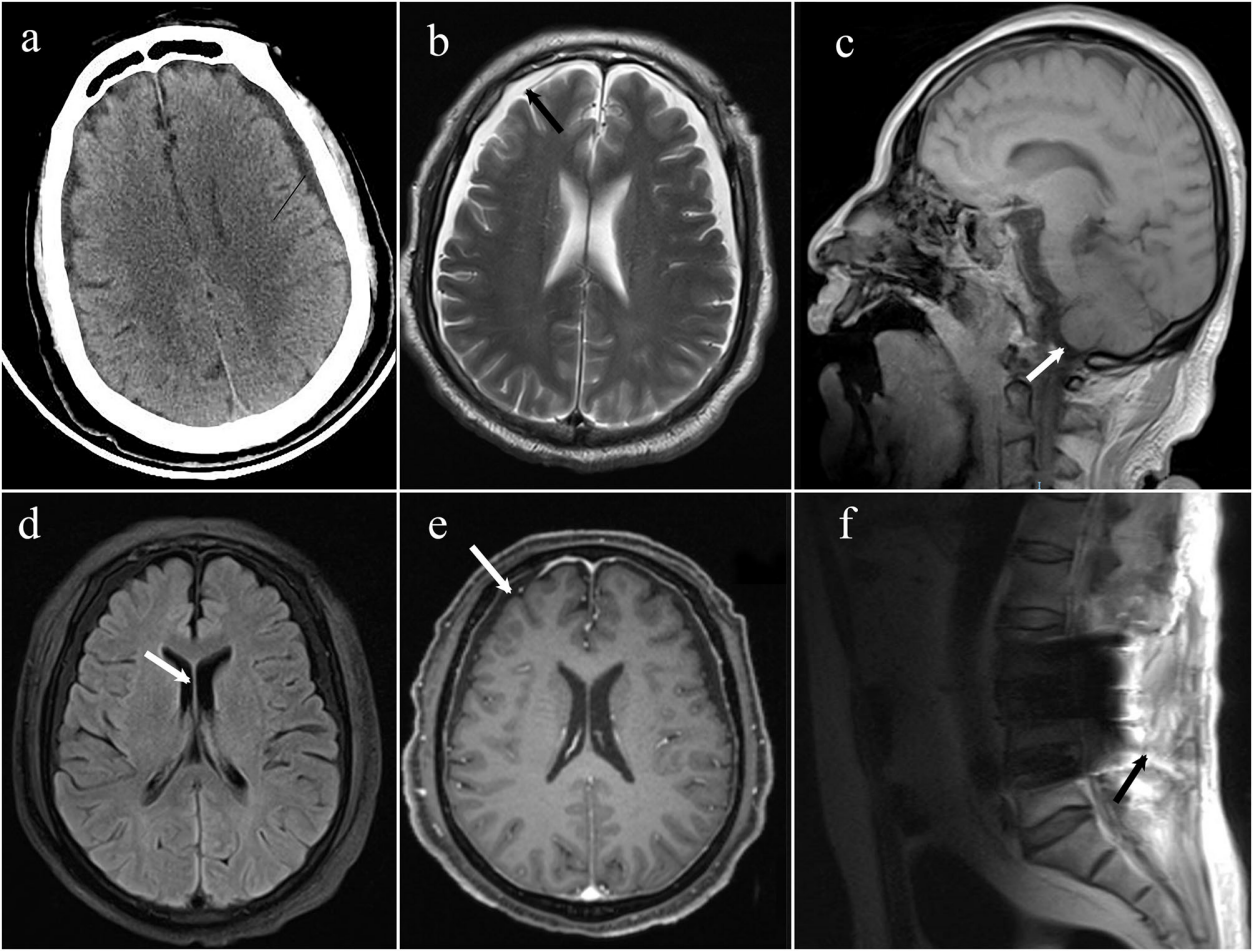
(a) The emergency CT suggested that the space under the inner plate of the skull was widened on both sides, which was considered a subdural effusion; (b) axial T2 weighted MRI demonstrates bilateral subdural effusions (arrows); (c) sagittal T1-weighted MRI demonstrates sagging of the brain; (d) axial T1 weighted MRI demonstrates bilateral ventricles narrowing; (e) axial T1 post-gadolinium showing diffuse, mild pachymeningeal thickening, and enhancement (arrow); (f) MRI spine sagittal T2 sequence demonstrates a fluid collection within the posterior paraspinal soft tissue.
